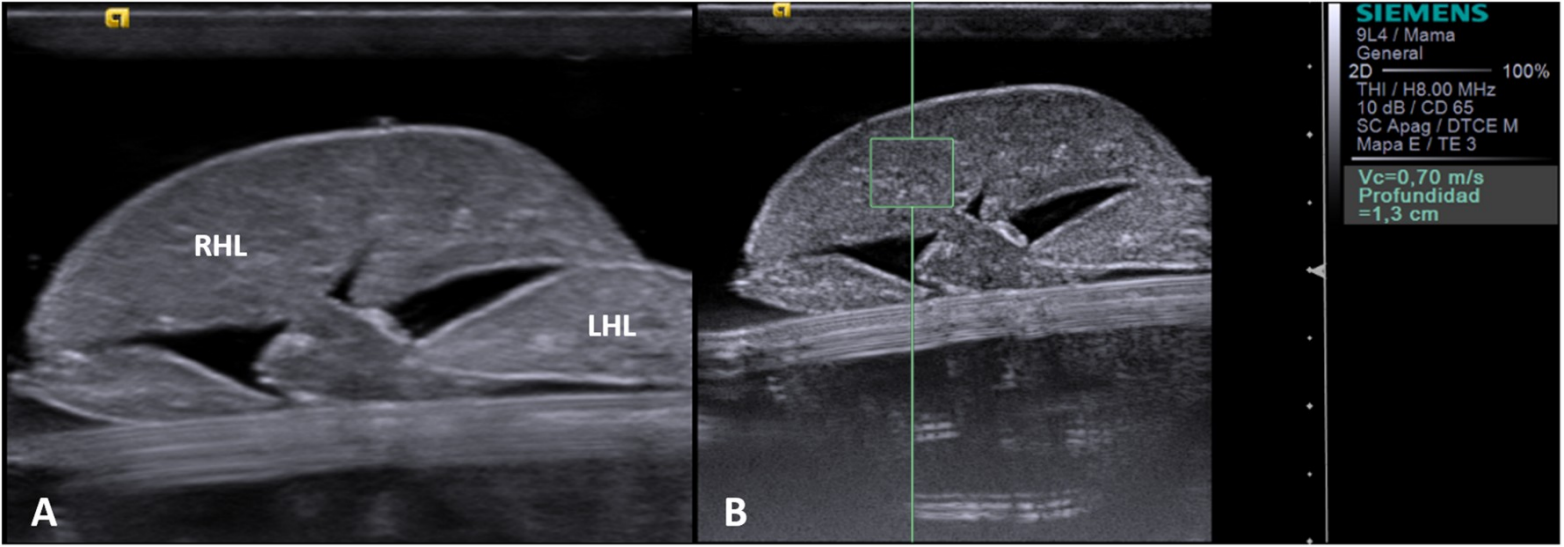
A, *Ex vivo* ultrasound image of the liver explant. RHL: right hepatic lobe; LHL: left hepatic lobe. B, *Ex vivo* assessment using ARFI.
